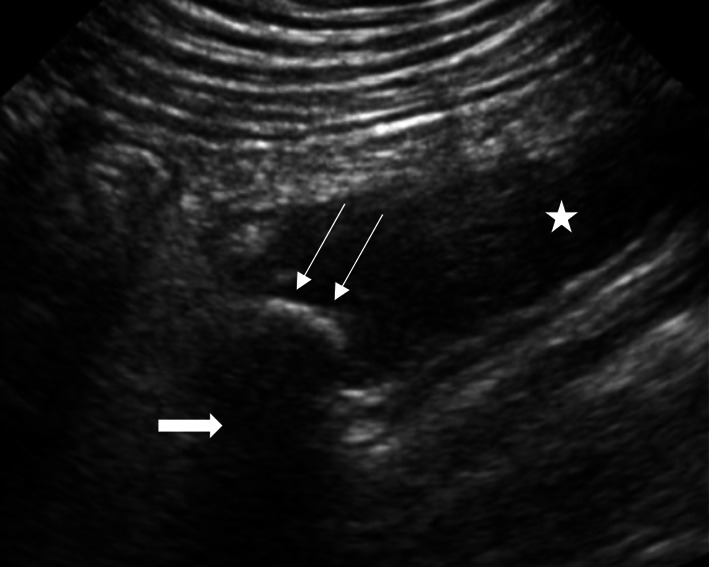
Appendicolith: Ultrasound abdomen of the right lower quadrant showing an appendicolith (long thin arrows), which is the echogenic area within the appendix lumen (distal appendix) (star). There is a posterior acoustic shadowing seen behind the echogenic foci (thick arrow). The largest appendix diameter measured was 16 mm
